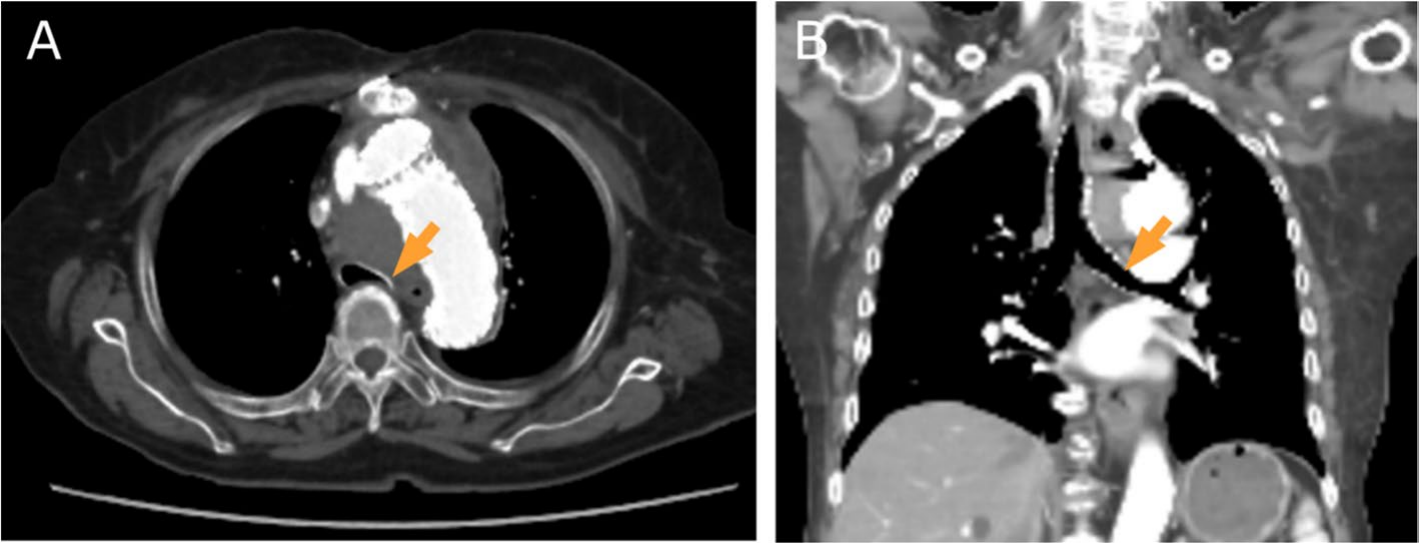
CT image of the chest on day Z + 7 (A transverse plane, B coronal plane). There is improvement of the tracheal compression (arrows)
